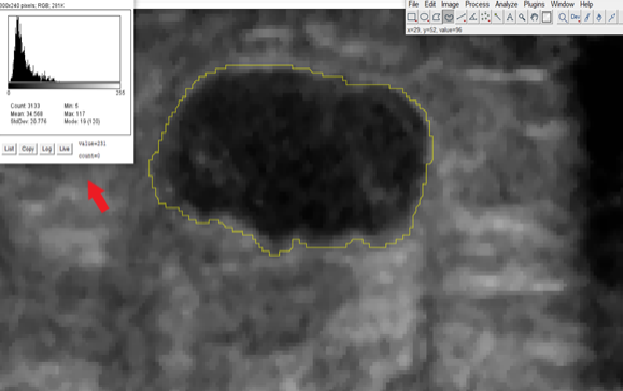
Despeckled ultrasound image of a hypoechoic malignant breast lesion. The image shows the freehand region of interest drawn over the lesion using ImageJ software. Gray scale intensity histogram (red arrow) of the lesion showing the mean, median, and SD values.
